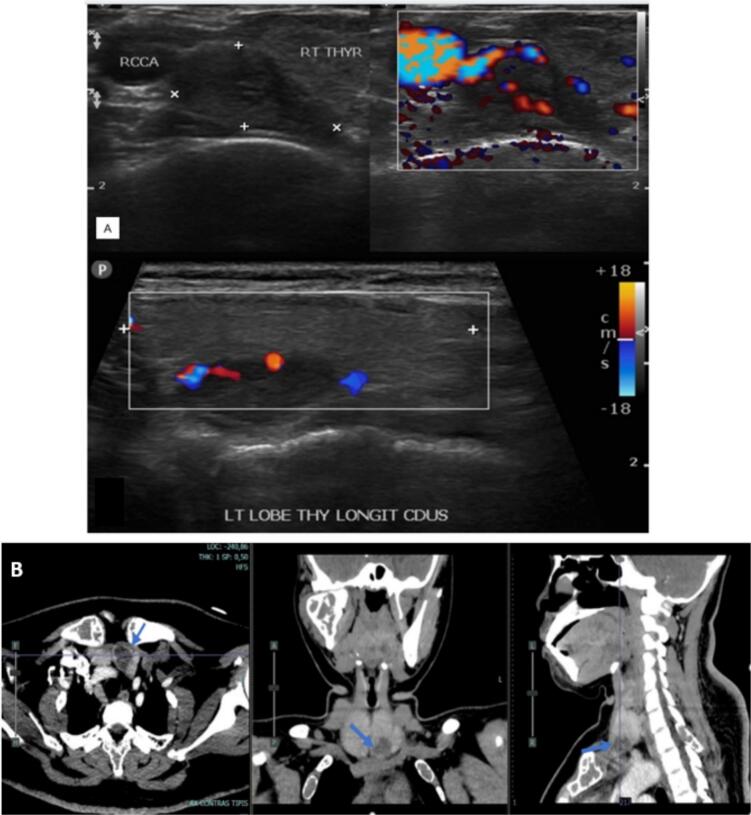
Ultrasound image of a patient with brown tumor, showing a hypoechoic lesion in the parathyroid gland consistent with the diagnosis (A), cervical CT scan with contrast revealed a solid mass in the posteroinferior aspect of the left thyroid lobe, supporting the parathyroid mass (B).
